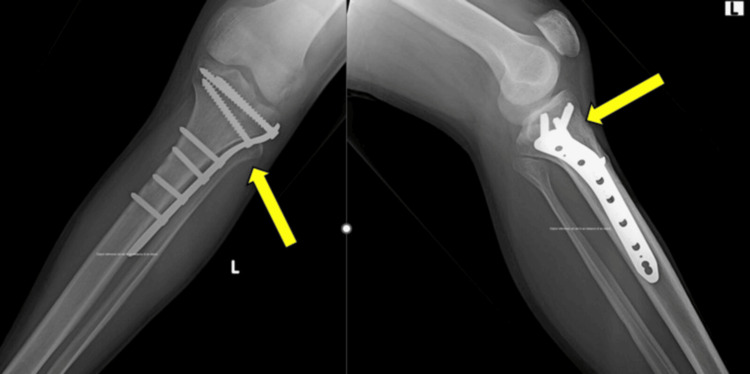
Immediate postoperative radiographs showing fixation with proximal tibial locking compression plate using minimally invasive percutaneous plate osteosynthesis technique.
